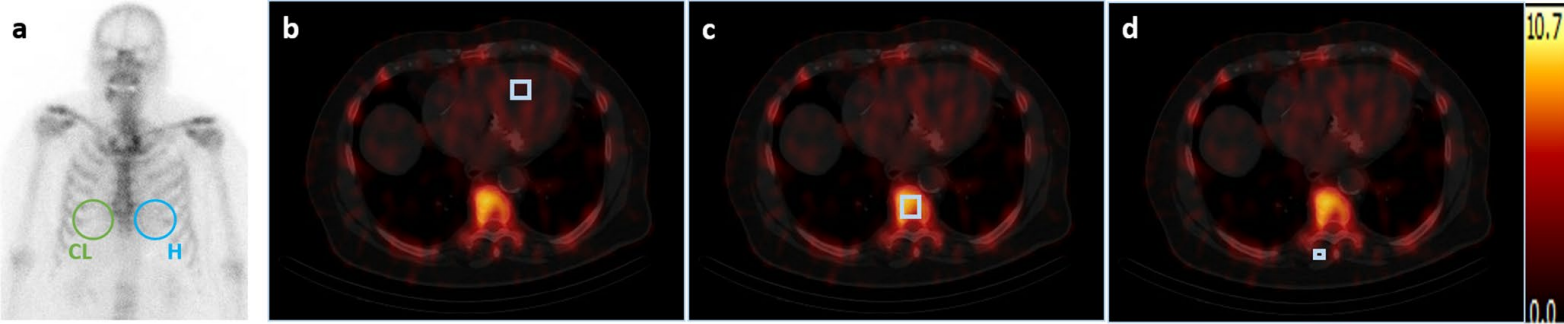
Nuclear medicine imaging analysis. Whole-body planar images were analysed semi-quantitatively by deriving the H/CL ratios (a). LV myocardial [^99m^Tc]-DPD-uptake was quantified on the SPECT/CT images as peak standardised uptake value (SUV_peak_) by placing manually cubic VOIs within the myocardial tissue of the interventricular septum (2 ml) (b), on the 8th thoracic vertebra (2.92 ml) (c) as well as on the left paraspinal muscle (1.19 ml) (d), respectively. The tracer retention index was additionally derived. [^99m^Tc]-DPD, [^99m^Tc]−3,3-diphosphono-1,2-propanodicarboxylicacid; H/CL, heart-to-contralateral; SPECT/CT, single photon emission computed tomography/computed tomography; VOIs, volumes of interest
